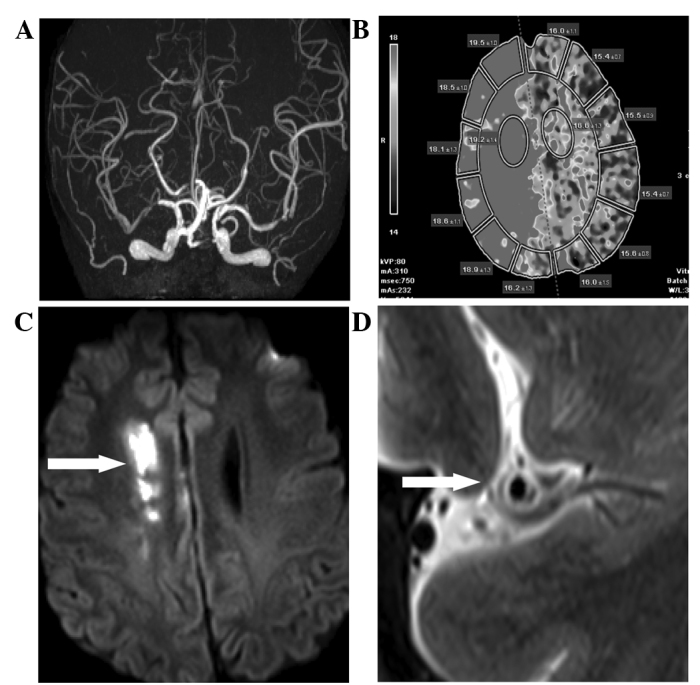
A 71-year-old female patient presented with dysarthria and mild left hemiparesis. (A) MRA from the right proximal MCA (M1 segment) showed a focal severe stenosis. (B) DWI confirmed internal border-zone infarction, which corresponded to the right MCA territory with the underlying mechanism of hypoperfusion and impaired emboli clearance. (C) Computed tomography perfusion imaging also demonstrated relatively low cerebral blood flow, and elevated time-to-peak in a region of the right MCA indicated the potential of hypoperfusion. (D) The T2-weighted HRMRI cross-sectional lumen image showed a hyposignal eccentric core surrounded by a high-signal thickened wall. The arrows represent the position of the lesion. MRA, magnetic resonance angiography; MCA, middle cerebral artery; DWI, diffusion-weighted imaging; HRMRI; high-resolution magnetic resonance imaging.
